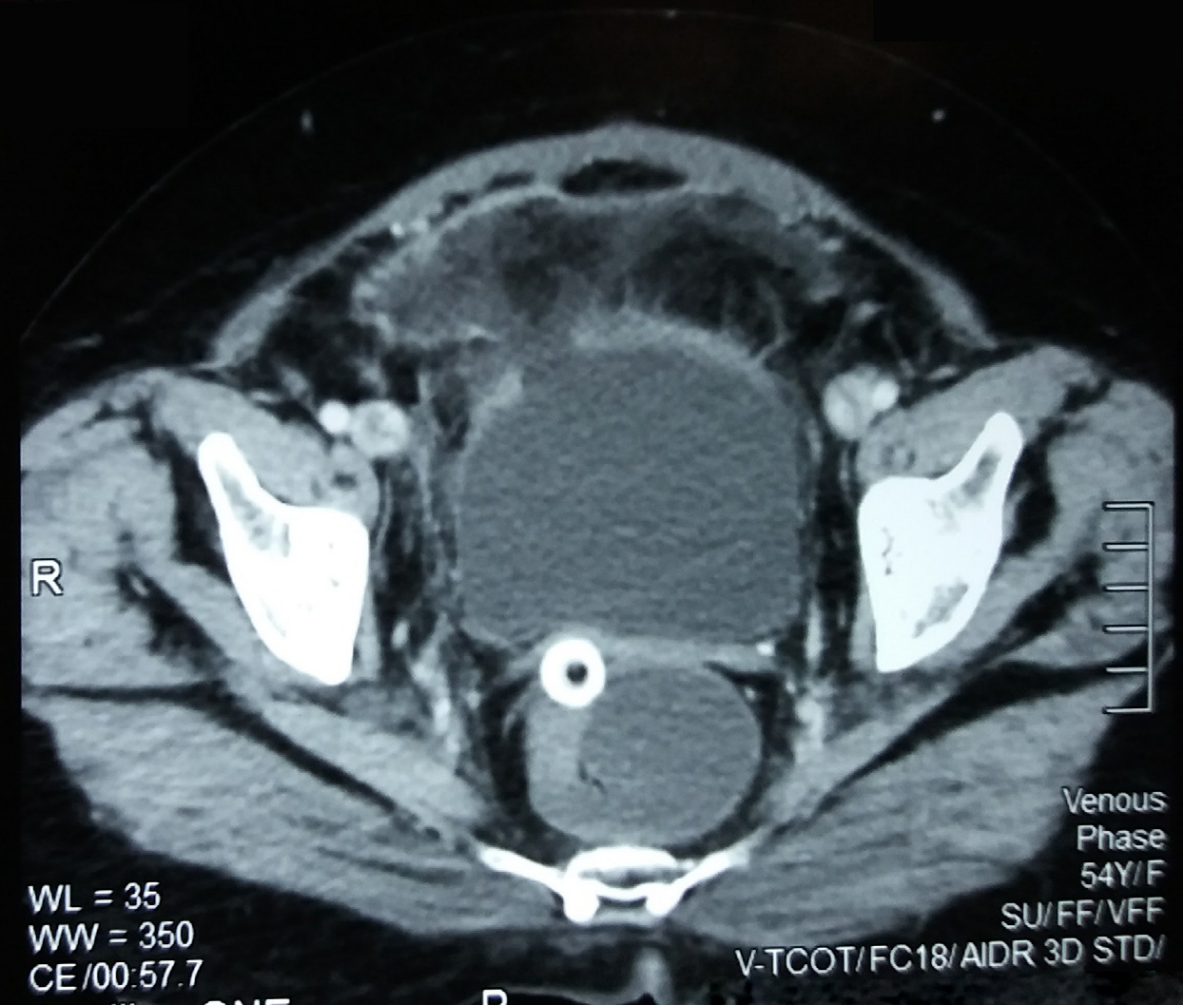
CT scan (Computed tomography) of the abdomen showing a 5.0 cm (Anteroposterior) × 7.0 cm (Transverse) × 7.0 cm (craniocaudal) space‐occupying lesion in the anterior wall of the bladder and the anterior abdominal wall with adjacent irregularly thickened bladder wall showing contrast enhancement.
